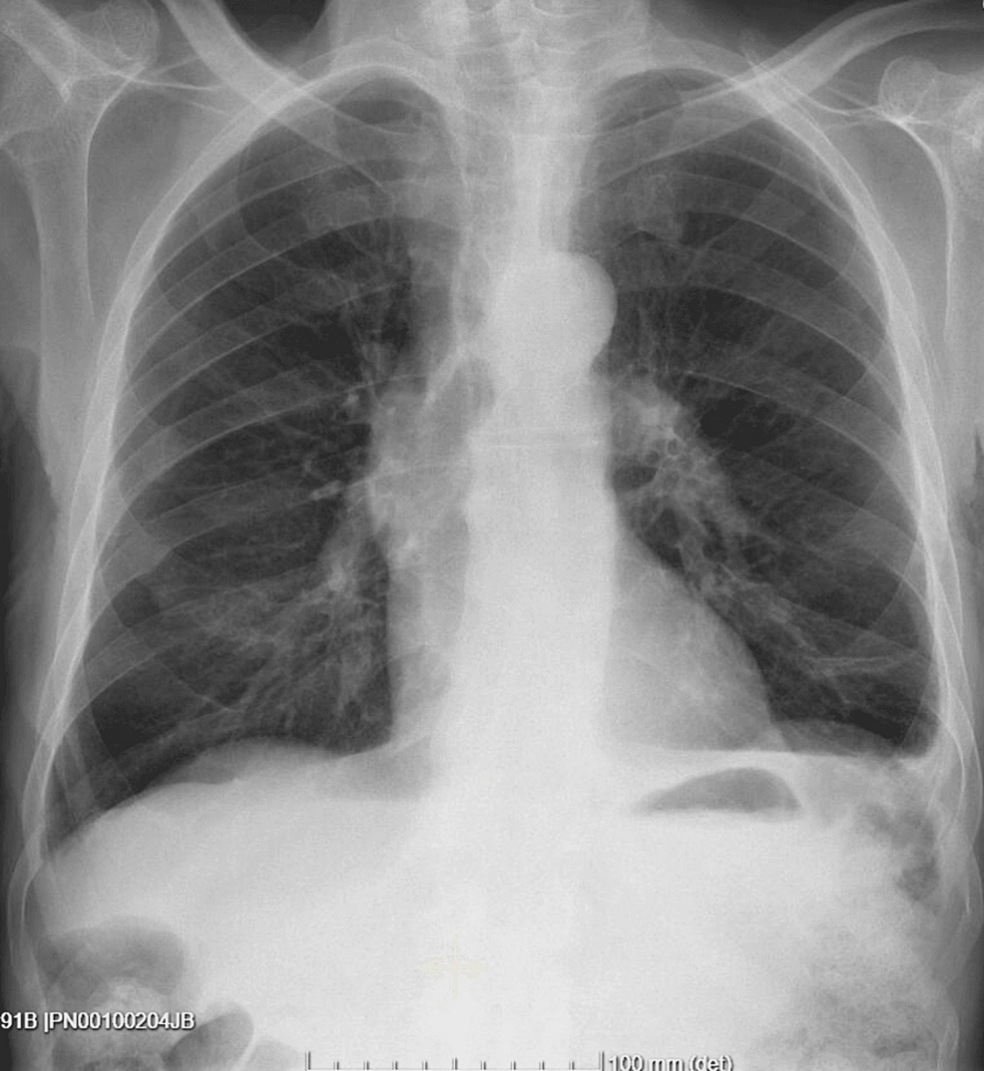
Chest X-ray at the time of discharge
